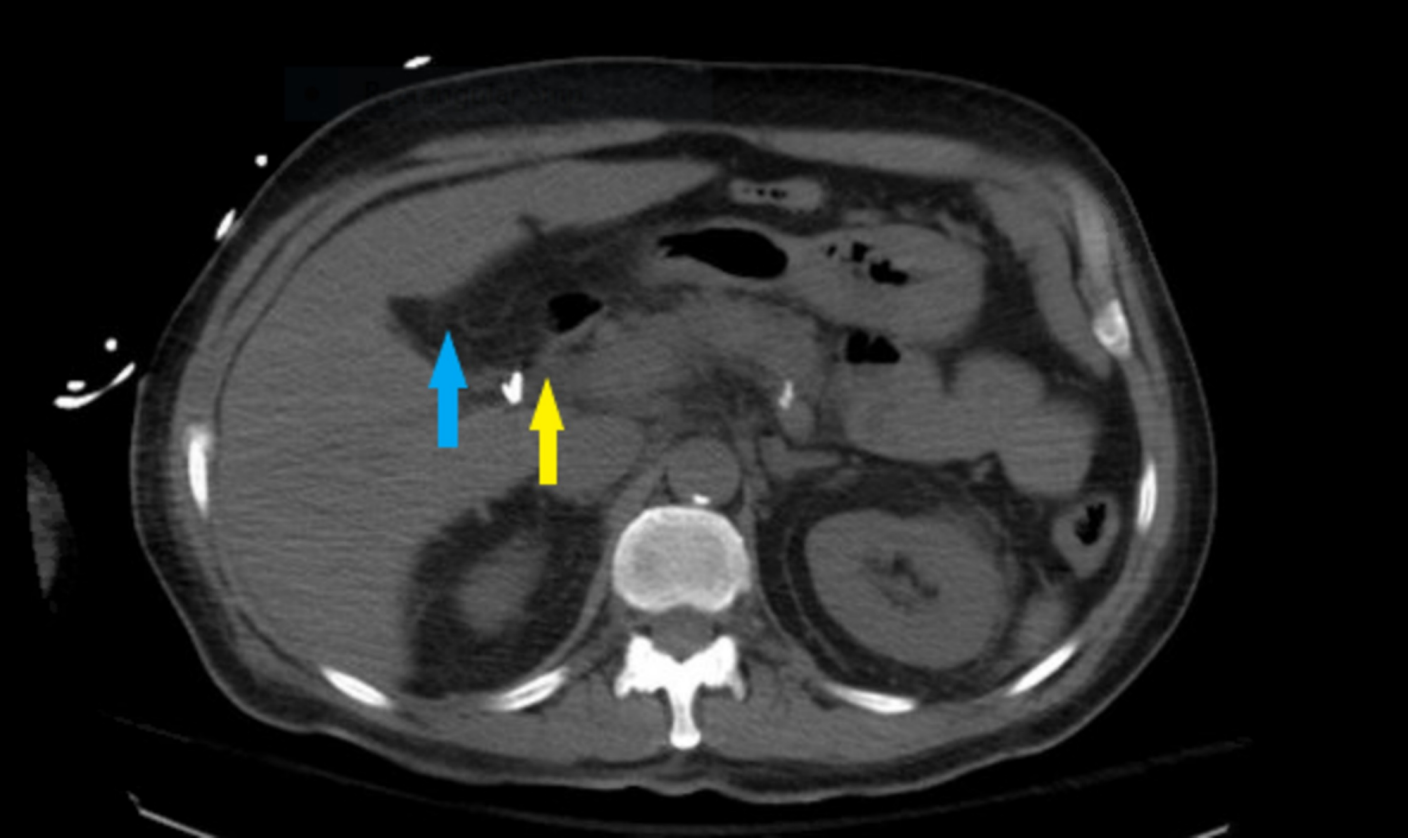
CT scan of the patient with intravenous contrast. CT scan reveals peripancreatic fat  stranding (yellow arrow). The absence of the gallbladder can be noted in the gallbladder fossa as the patient had a cholecystectomy in the past (blue arrow).
